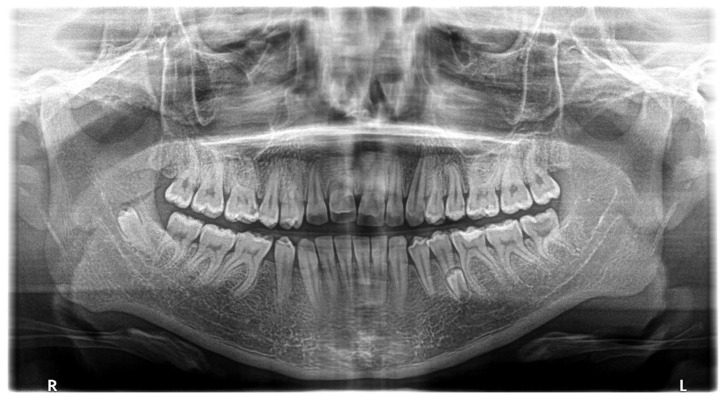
Panoramic radiograph, taken four years later, when the patient was 20 years old, showing the germ of the tooth 45, which was found in the ramus of the mandible above the mandibular canal at the same height as the crowns of the lower teeth. The fourth additional tooth had developed and located itself in the projection of 1/3 of the apical part of the root of the tooth 35. Additionally, a resorption of the root of the tooth 11 became noticeable.
